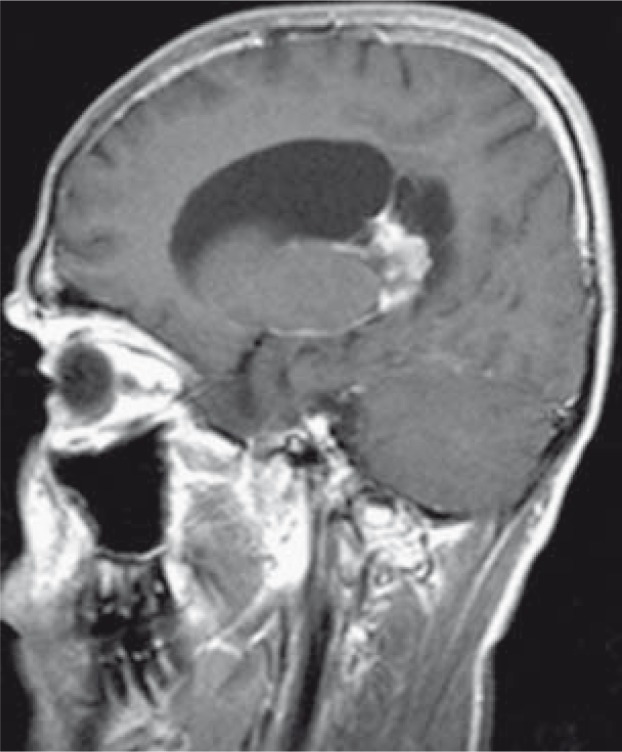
Axial T1 post-contrast MR image shows choroid plexus papilloma in the lateral ventricle.
